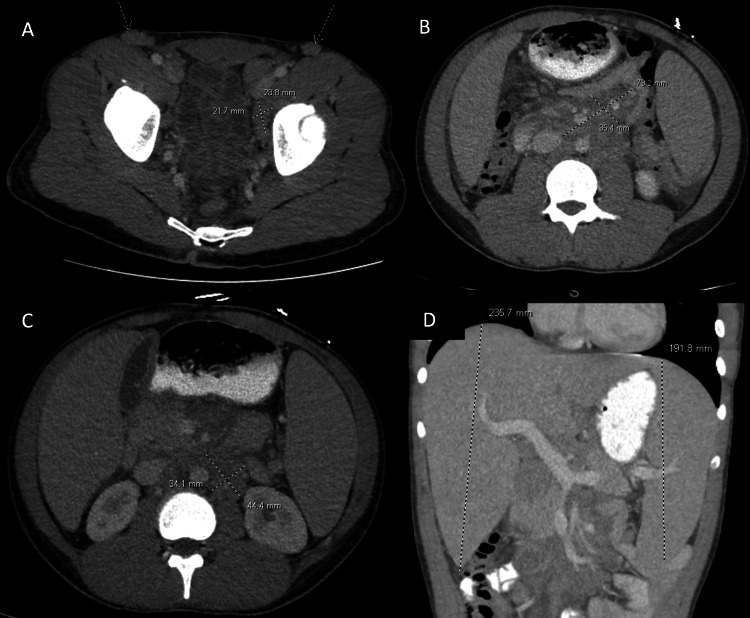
Case 1: computed tomography (CT) showing lymphadenopathy and hepatosplenomegaly Mesenteric and retroperitoneal lymphadenopathy. Axial and coronal intravenous contrast material–enhanced CT image shows (A) left external iliac nodal mass measuring 2.88 x 2.17 mm (dashed cross) and bilateral inguinal lymphadenopathy (dashed arrows). (B) A central mesenteric conglomerate nodal mass measuring 7.32 x 3.54 mm (dashed cross). (C) Left para-aortic nodal mass measuring 4.44 x 3.41 mm (dashed cross). (D) Hepatosplenomegaly (dashed lines).
